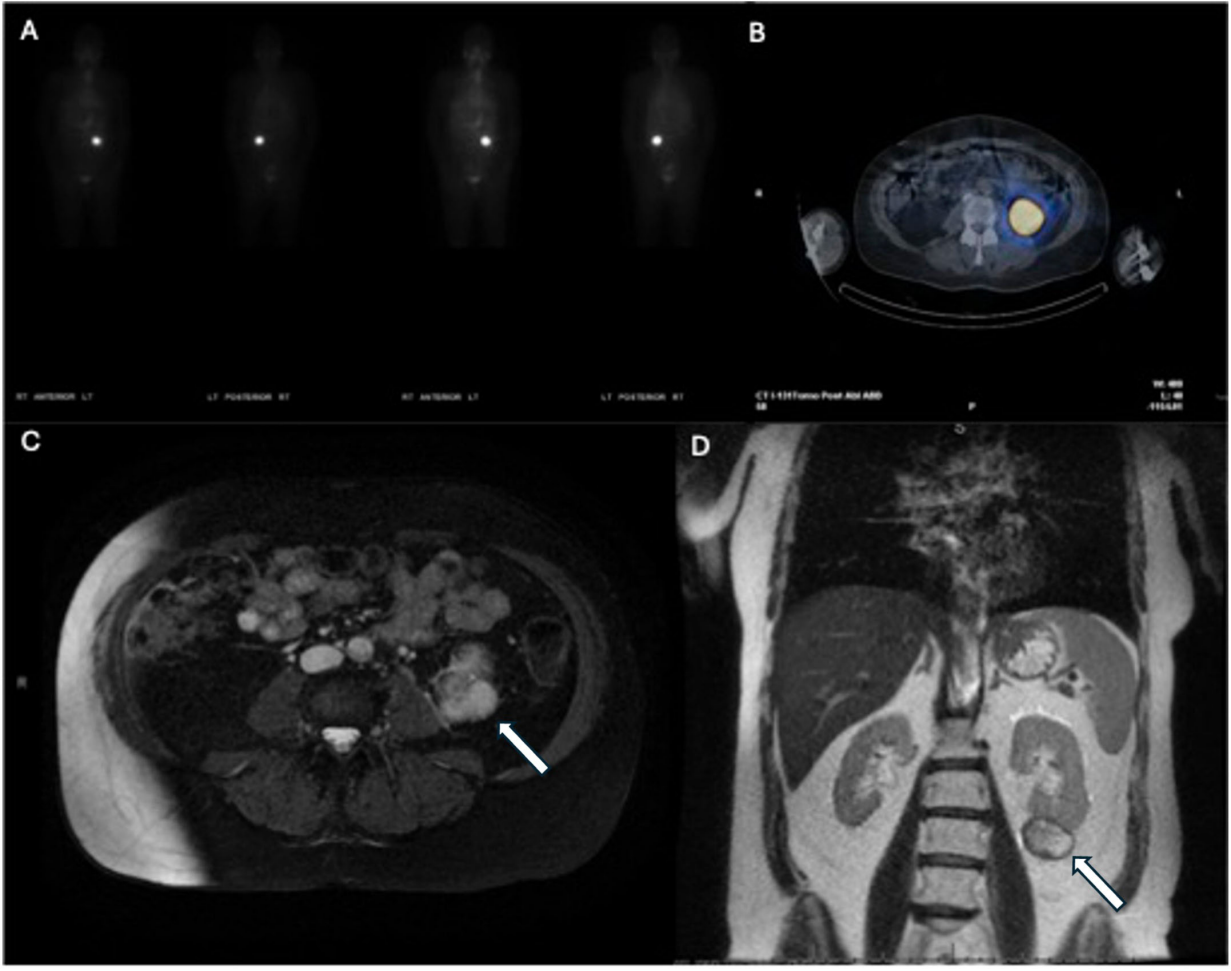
Post-radioactive iodine ablation I-131 whole-body scan (A) and axial view (B) demonstrating increased uptake in the area of the left kidney. Subsequent T2-weighted axial (C) and T1-weighted coronal (D) MRI demonstrating the left kidney mass (arrows).
